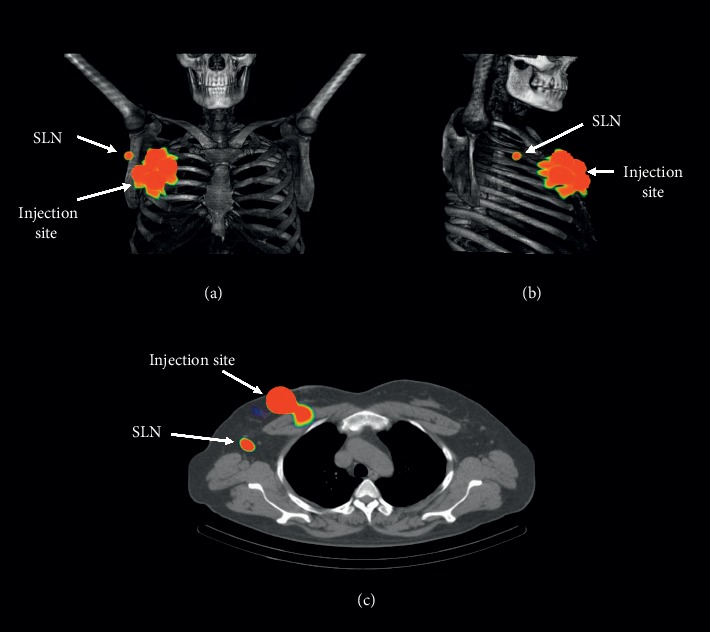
SPECT/CT imaging of the patient number 1 after 6.5 h of ^99m^Tc-AuNP-mannose (37 MBq) administration. (a) Frontal and (b) lateral views. (c) Slice of the fused SPECT/CT imaging.
